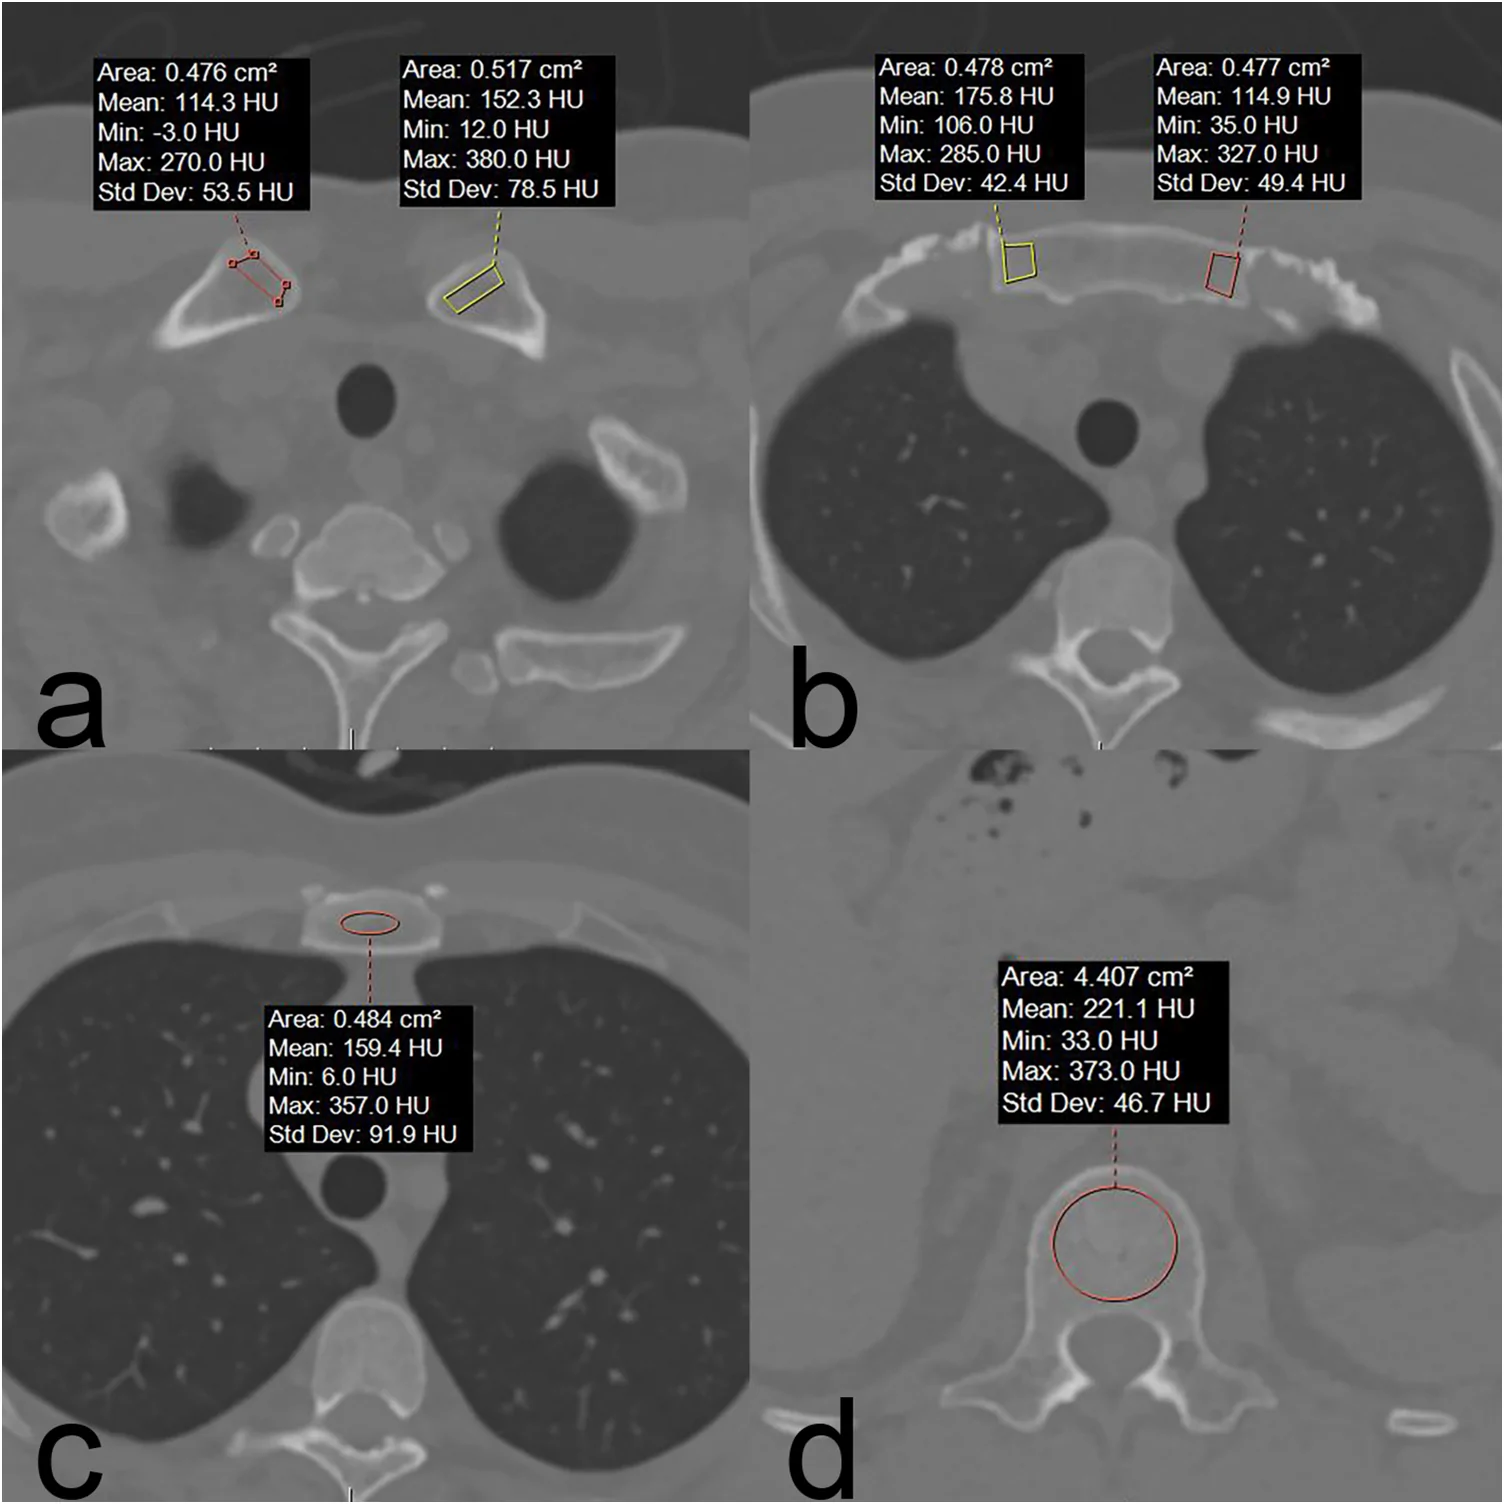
Representative measurement of CT attenuation values at the five anterior chest wall sites and the reference vertebra. Regions of interest (ROIs) were placed on axial CT images at the following sites: (a) left and right clavicular heads; (b) left and right upper manubrium at the level of the first sternocostal joint; (c) sternal angle; and (d) reference vertebra. L1 was preferred as the reference vertebra; when L1 was unsuitable because of an abnormality affecting cancellous bone attenuation, a structurally normal adjacent T12 or L2 vertebra was used. Quantitative measurements were performed on the B70f reconstruction series. The images are displayed using a wide bone-window setting (window width 4000 HU; window level 200 HU). The same measurement protocol was applied to all participants.
